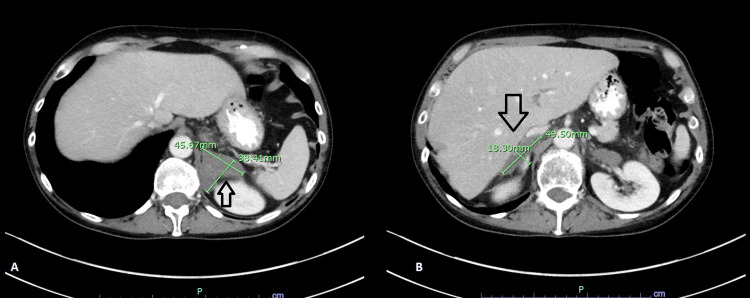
CT Abdomen A follow-up CT abdomen showed a slight reduction in the size of adrenal metastases (arrows in A and B).
